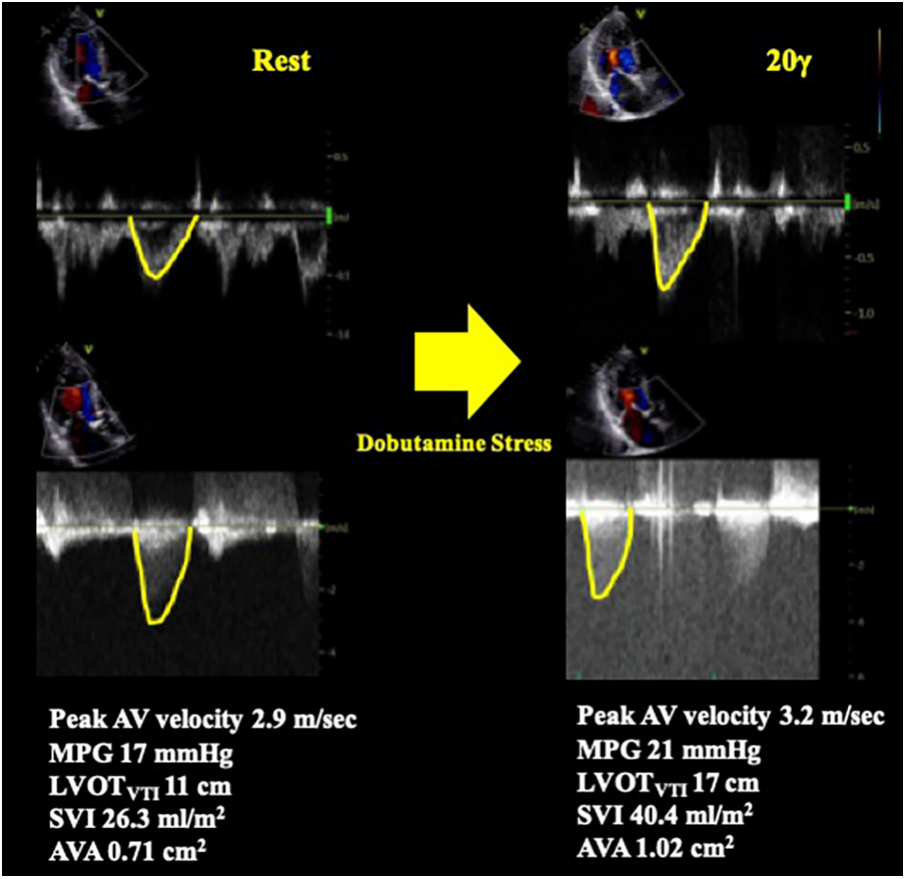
Calculation of projected aortic valve area with dobutamine stress echocardiography. AVA, aortic valve area; FR, flow rate; SV, stroke volume.
